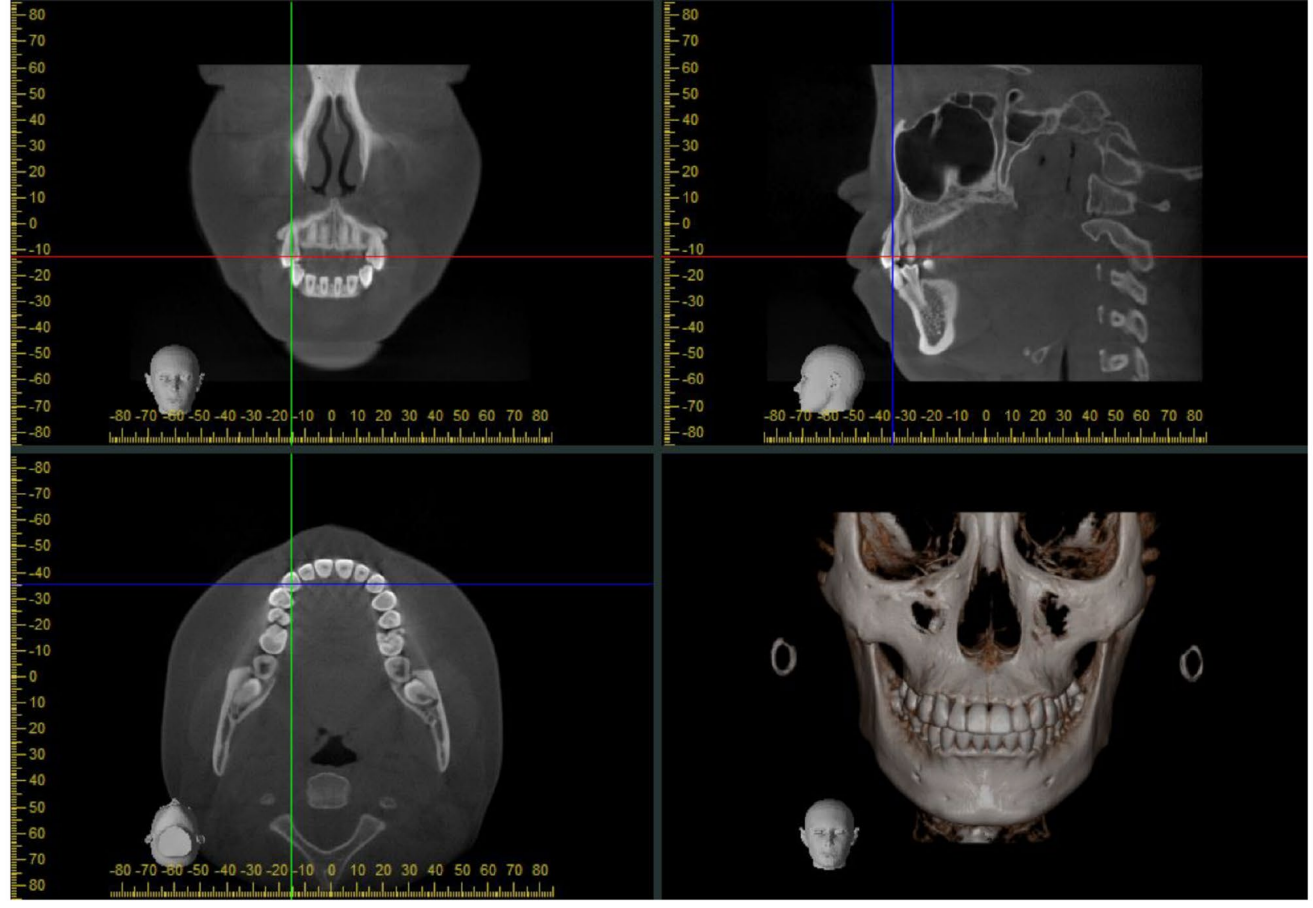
Three-dimensional image presentation of CBCT. The green guideline adjusts the sagittal orientation. The red guideline adjusts the axial orientation. The blue guideline adjusts the coronal orientation
